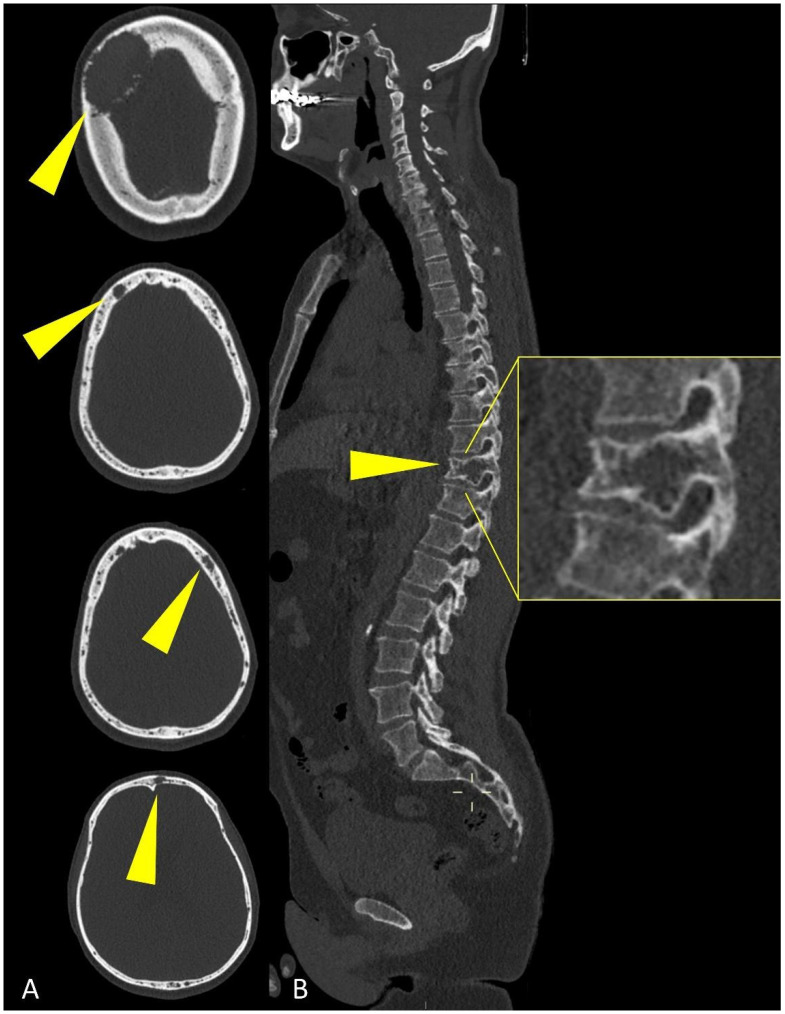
Multiplanar CT images showing (yellow arrowheads) osteolytic lesions of (A) the skull and of (B) a dorsal vertebra (detail in (B)).
